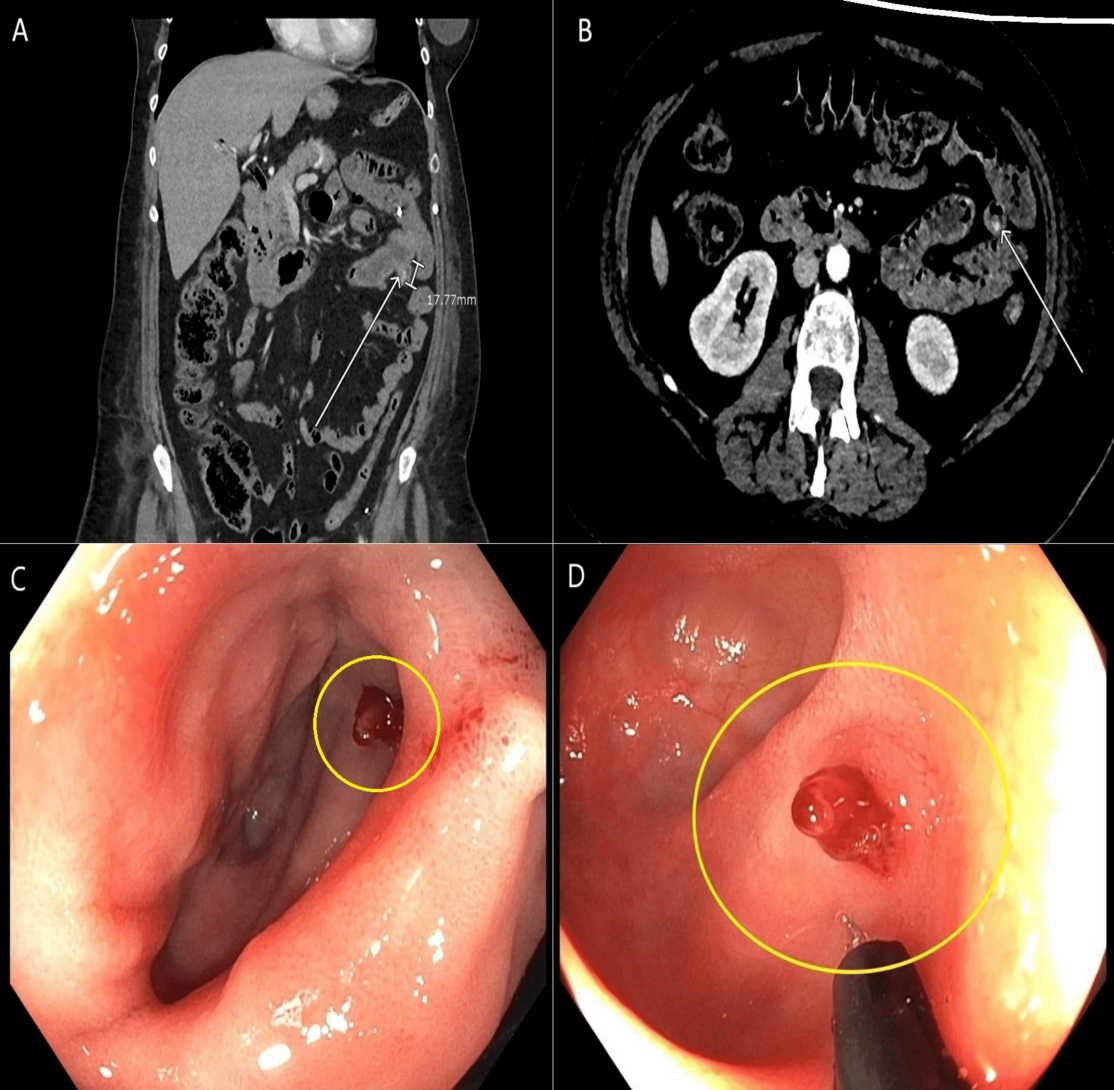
Imaging and endoscopic findings. (A) CTA of the abdomen and pelvis with contrast, coronal view, demonstrating approximately 1.8 cm jejunal diverticulum (white arrow). (B) CTA of the abdomen and pelvis with contrast, axial view, arterial phase, concerning for active extravasation (white arrow). (C, D) EGD and push enteroscopy with a visible vessel inside a large jejunal diverticulum (yellow circles). CTA: computed tomography angiography; EGD: esophagogastroduodenoscopy
